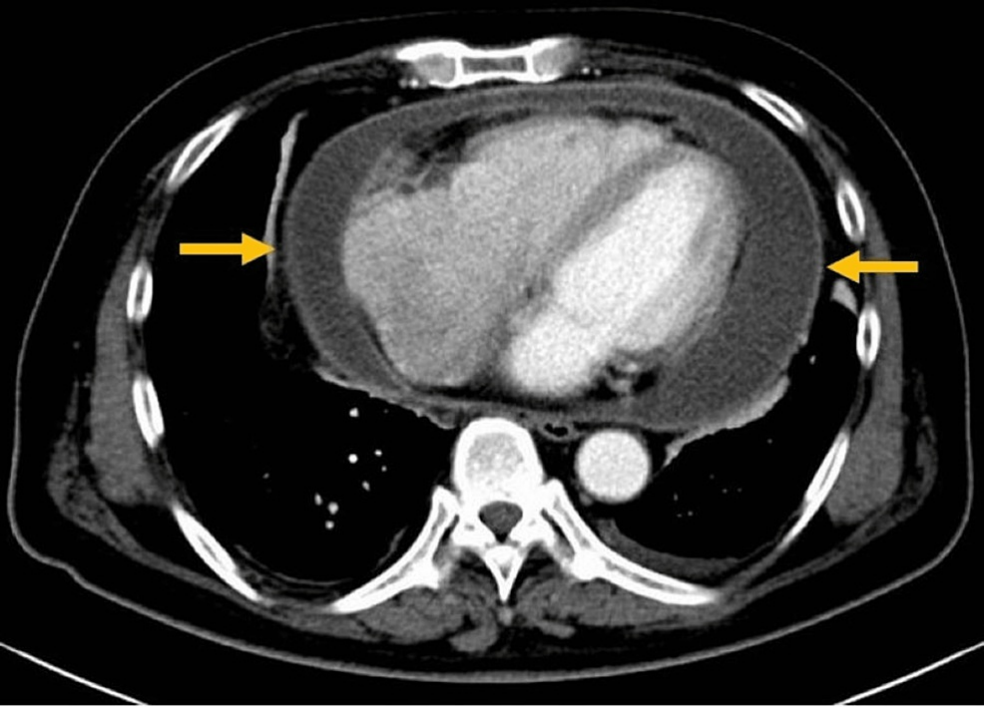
CT scan of chest on admission showed pericardial effusion CT: computed tomography
